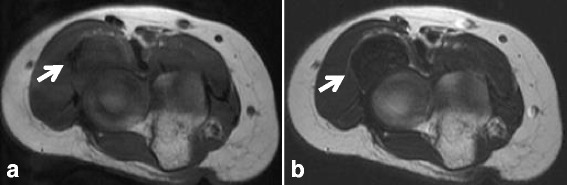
T1- and T2-weighted MR images of Case 1. a On T1-weighted images, the mass (arrow) is isointense compared to muscle. b On T2-weighted images, the mass (arrow) has low signal intensity compared to muscle
